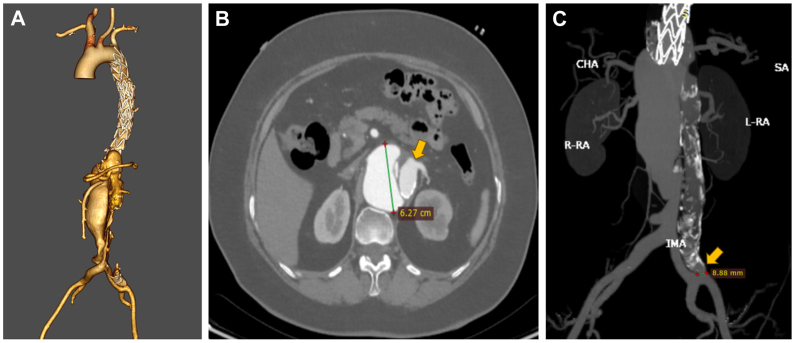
(A) Computed tomography angiography (CTA) revealed a postdissection thoracoabdominal aortic aneurysm, with the celiac trunk and left renal artery arising entirely from the false lumen. (B) CTA demonstrated a postdissection thoracoabdominal aortic aneurysm measuring 62.7 mm in diameter. The *arrow* indicates the left renal artery arising entirely from the false lumen. (C) CTA demonstrates an 8.9-mm entry tear in the left common iliac artery communicating with the false lumen (*arrow*).
